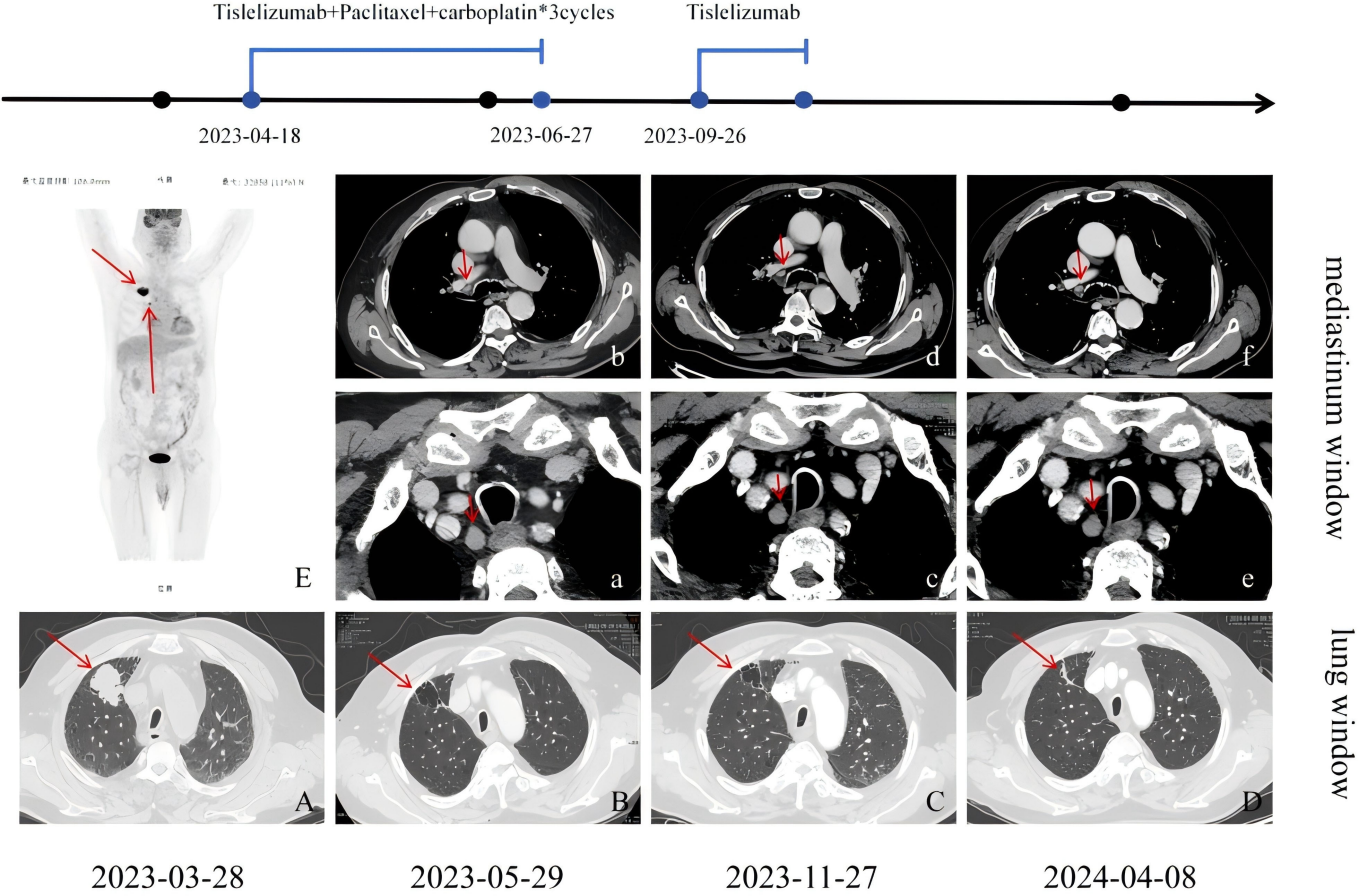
(A) Irregular soft tissue shadows are seen in the anterior segment of the upper lobe of the right lung, with lobulated and spiculated margins; (B) Following treatment for right upper lobe lung cancer, there was an irregular thin-walled cystic lesion measuring approximately 32×25×27 mm, with irregular linear septations and sparse lamellar dense shadows along the inner edge of the cyst; (C) The cyst was reduced in size compared to before, measuring approximately 31×20×22 mm, with almost complete collapse compared to before, presenting as cord-like dense shadows. (E) The FDG metabolism in the hilum of the right lung and mediastinal lymph nodes was increased to varying degrees, with the largest node having a diameter of approximately 17 mm. In the mediastinum, nodes in regions 10R (b), 4R, and 2R (a) were enlarged with moderate enhancement, with the largest nodes in 10R and 2R measuring approximately 16×10 mm. Regions 10R (d), 4R, and 2R (c) also showed several enlarged nodes with moderate enhancement, similar in size to previous findings. Regions 10R (f), 4R, and 2R (e) showed several enlarged nodes with moderate enhancement, similar in size to previous findings.
